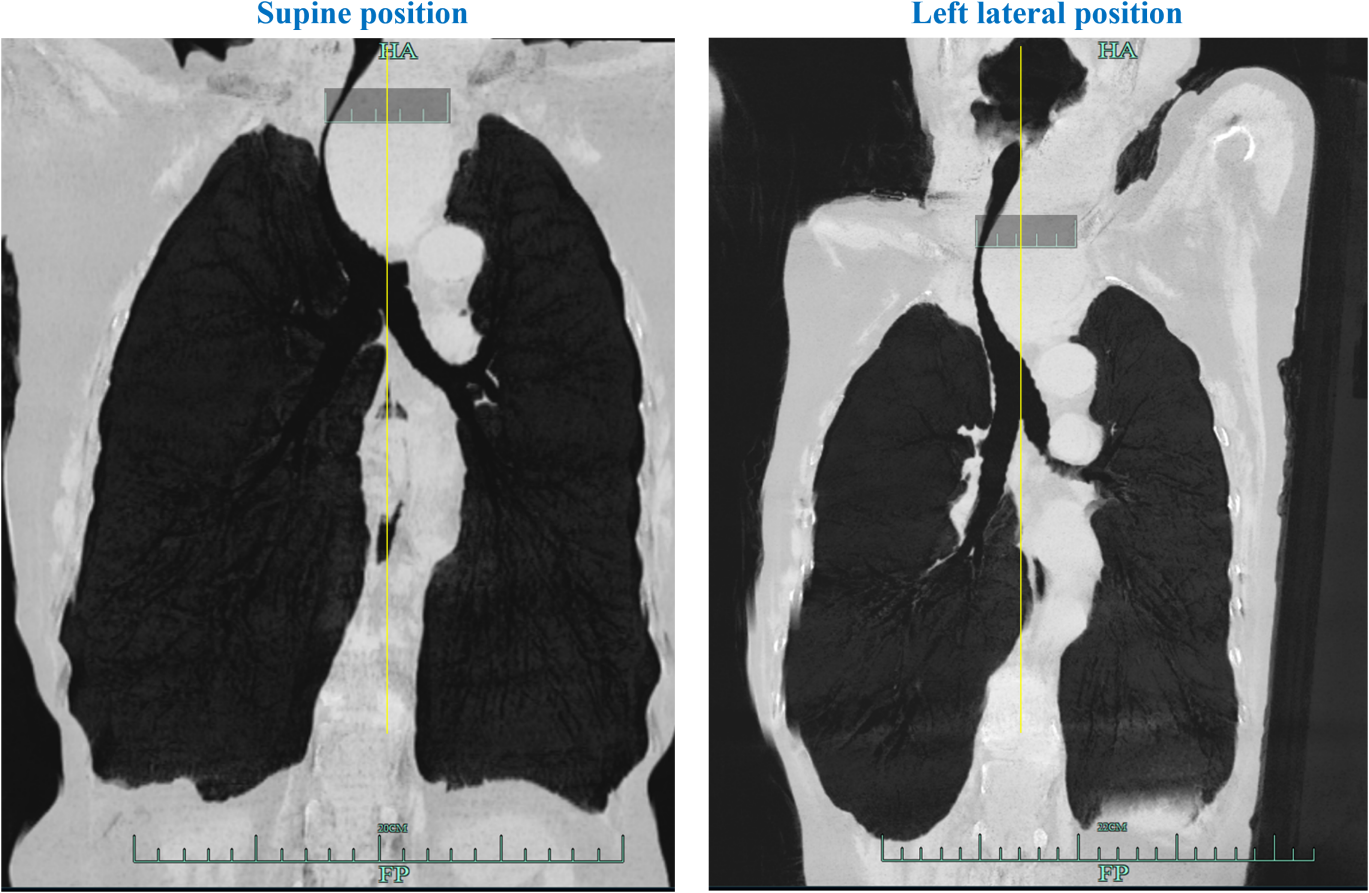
The imaging of coronal computed tomography (CT). The coronal CT scan of the airway showing that left lateral decubitus positioning results in an attenuated rightward tracheal deviation and a significant increase in the tracheal lumen diameter at its narrowest point.
